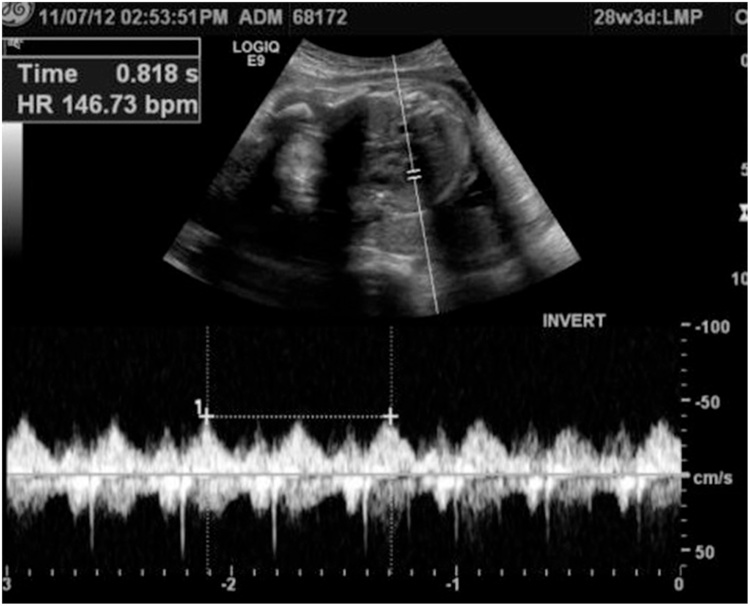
Fetal Ultrasound showed a healthy fetus.
